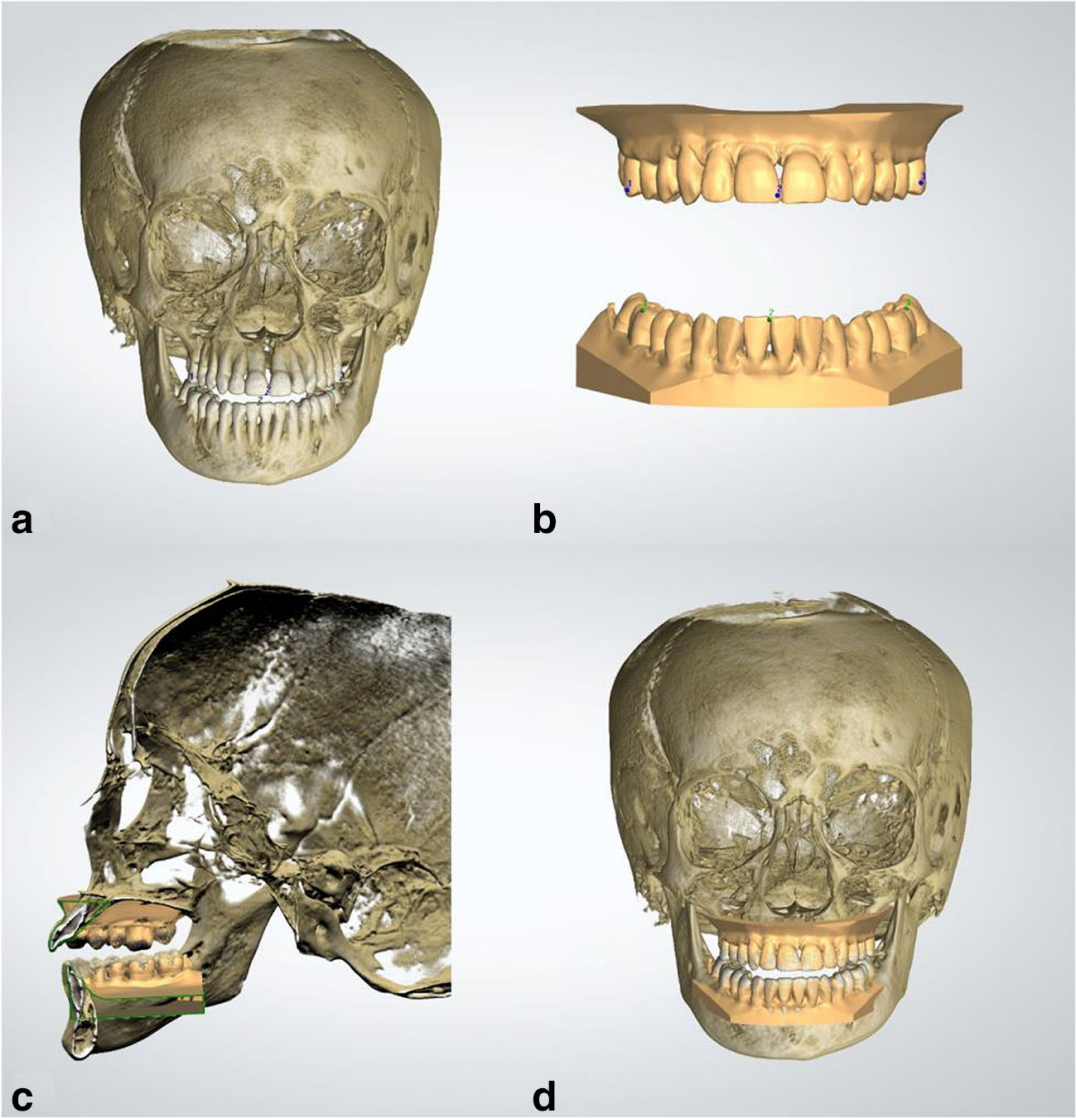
Overview of the fusion process of the intra-oral scans in the CBCT in OrthoAnalyzer™. a Three-dimensional CBCT skull model in OrthoAnalyzer™ with three points on both the maxillary and mandibular teeth. b Intra-oral scans of maxilla and mandible with three points on both the maxillary and mandibular teeth, corresponding with the points shown in 3A. c Cross-sectional view of the 3D CBCT skull model and fused intra-oral scans (shown in green). d Frontal view of the result of the fusion of the intra-oral scans in the CBCT
